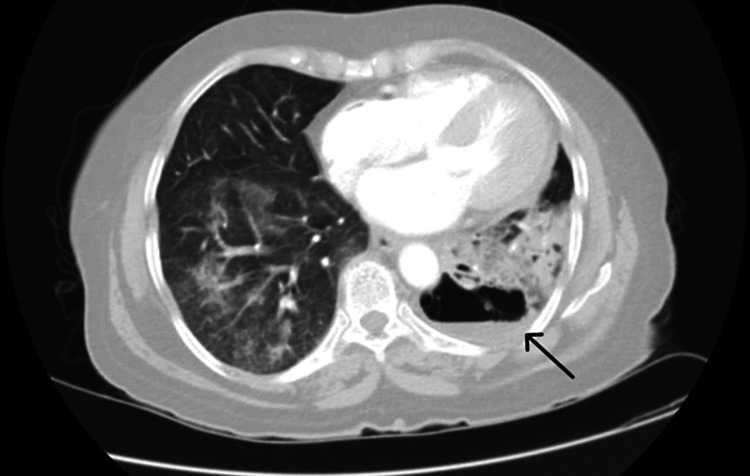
Contrast-enhanced computed tomography of the thorax showing consolidative changes with a lung abscess in the left lower lobe (black arrow)
